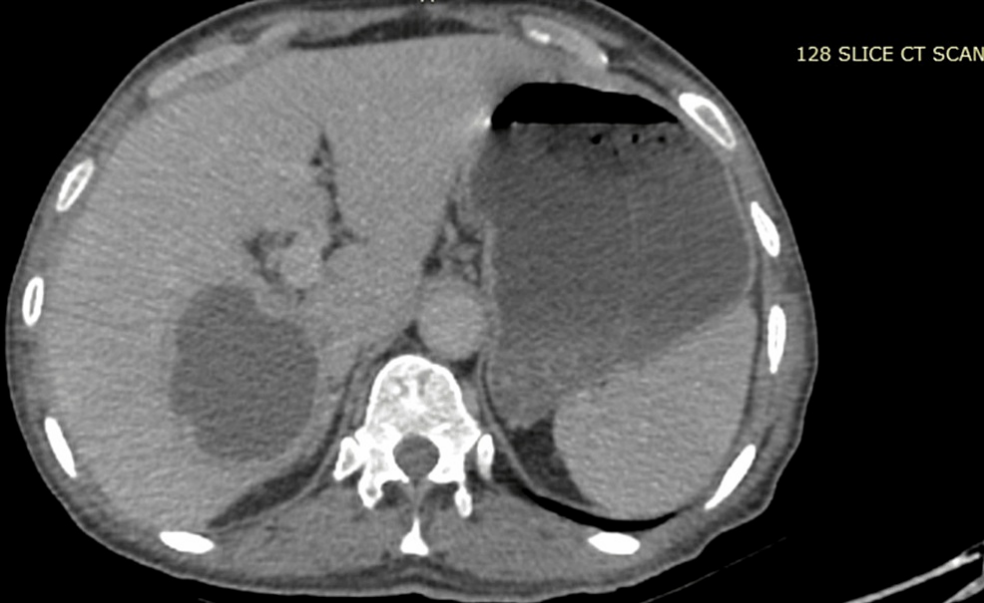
Axial section of contrast-enhanced CT Shows a well-defined hypodense lesion in segment 7 of the right lobe of the liver, with an internal non-enhancing area (suggestive of necrosis)
